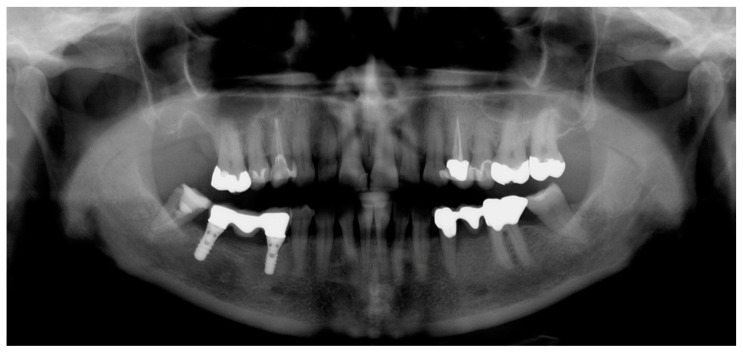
Panoramic radiograph (OPT).
